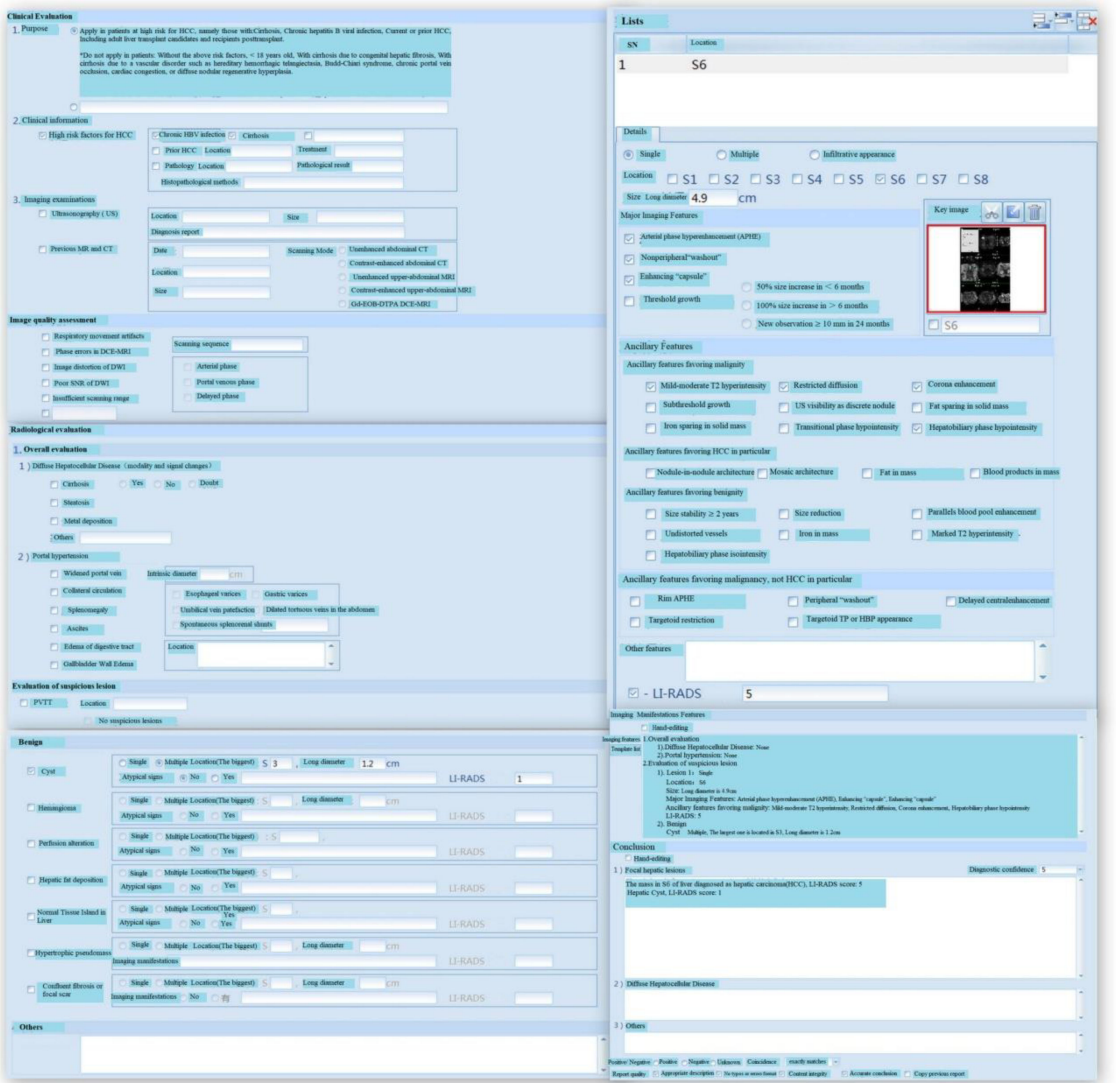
LI-RADS and Case Analysis. HBV, Hepatitis B Virus; Gd-EOB-DTPA, Gadolinium-Ethoxybenzyl-Diethylenetriamine penta-acetic acid; DCE, Dynamic Contrast Enhanced; DWI, Diffusion Weighted Imaging; SNR, Signal-to-Noise Ratio; PVTT, Portal Vein Tumor Thrombosis; HBP, Hepatobiliary Phase. LI-RADS is effective assessment tools for HCC and adopted by many clinical practices throughout the world. The score of LI-RADS can be calculated by filling in relevant contents according to the form.
